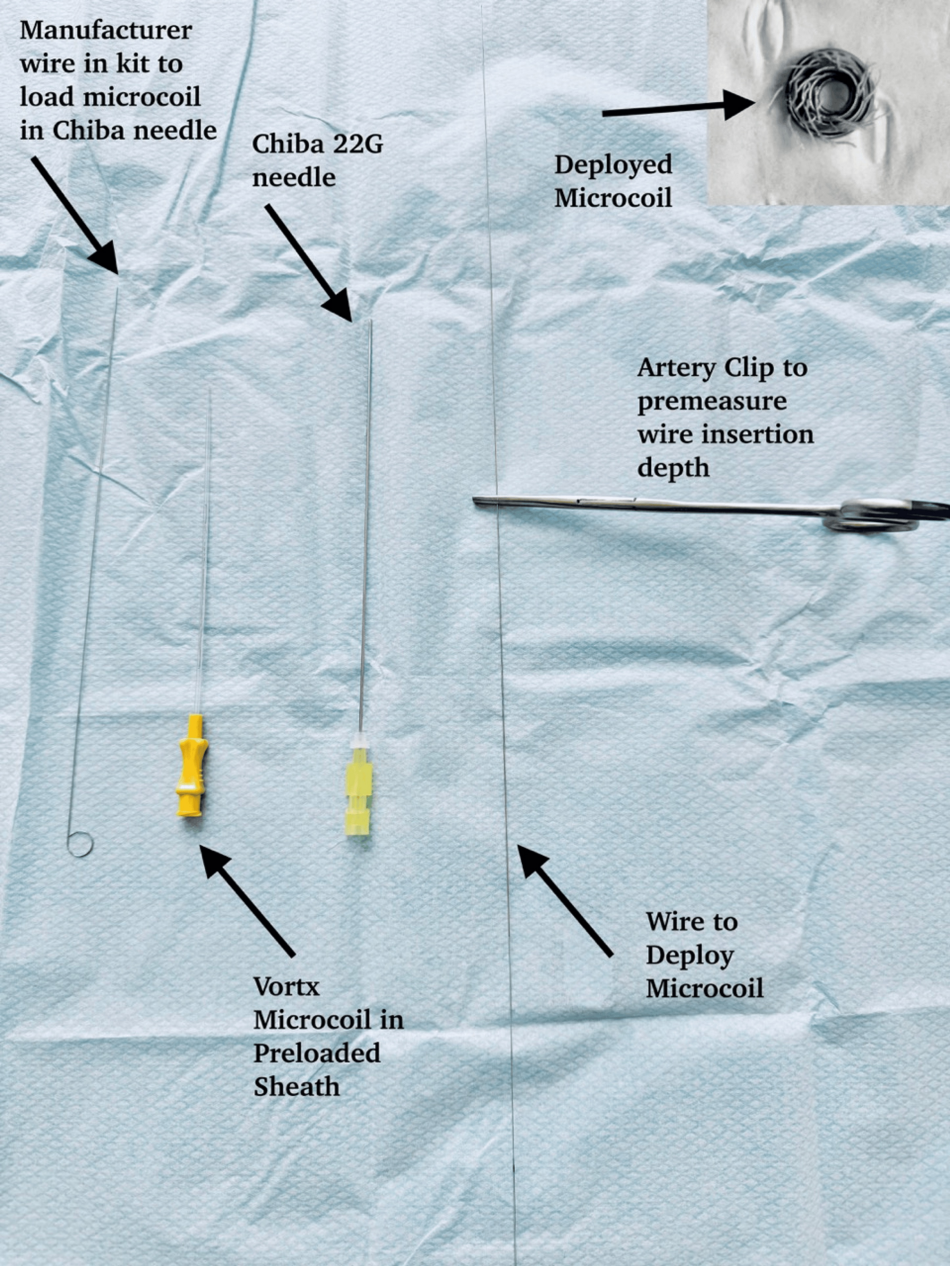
CT-guided pulmonary microcoil insertion equipment set-up
